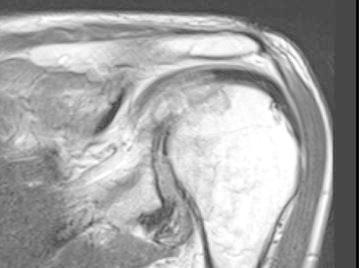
MRI of the shoulder showing moderate glenohumeral joint effusion with synovitis and large well-demarcated erosions on the humeral head
